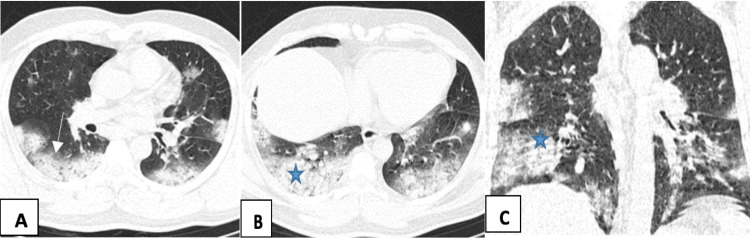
HRCT thorax axial and coronal reformatted images of 60-year-old male patient demonstrate a few patchy crazy pavement appearance (white arrow) and multiple peripheral areas of patchy consolidations (blue star), predominantly involving bilateral lower lobe with a CT severity score of 11/25 (moderate disease)
